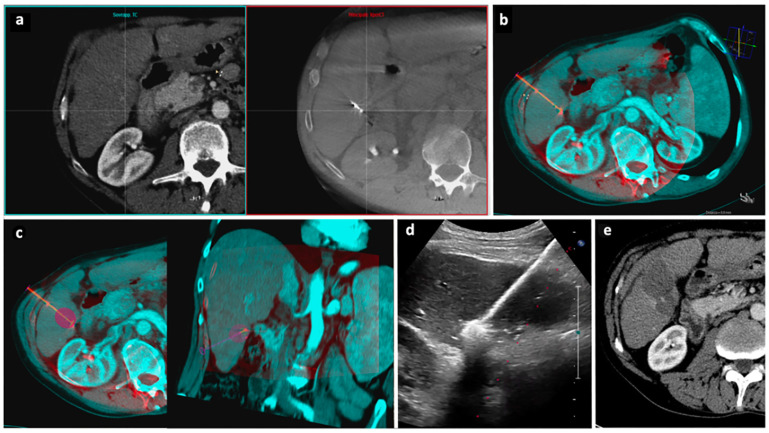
“Double fusion” technique—Part 2. After the MWA antenna is placed into the tumor (Figure 3), an unenhanced CBCT is acquired and CBCT fusion imaging is performed. (a) The manual registration process begins with the side-by-side visualization on the workstation of preprocedural CT images on the left and intraprocedural CBCT images on the right. (b) CT images are superimposed to CBCT images to assess the spatial relationship between the tumor and the MWA antenna; a virtual antenna is placed exactly over the real one. (c) The predicted ablation volume is displayed as a purple area at the tip of the virtual antenna based on the selected ablation power and time, according to the MWA device manufacturer data; the predicted ablation volume is here assessed on the axial (left) and coronal (right) planes. (d) If the antenna position is judged satisfactory by CBCT fusion imaging, ablation is carried out under US guidance. (e) Follow-up axial CT images acquired 1 month after procedure demonstrate complete response. Abbreviations—CBCT: cone beam computed tomography; CT: computed tomography; MWA: microwave ablation; US: ultrasound.
